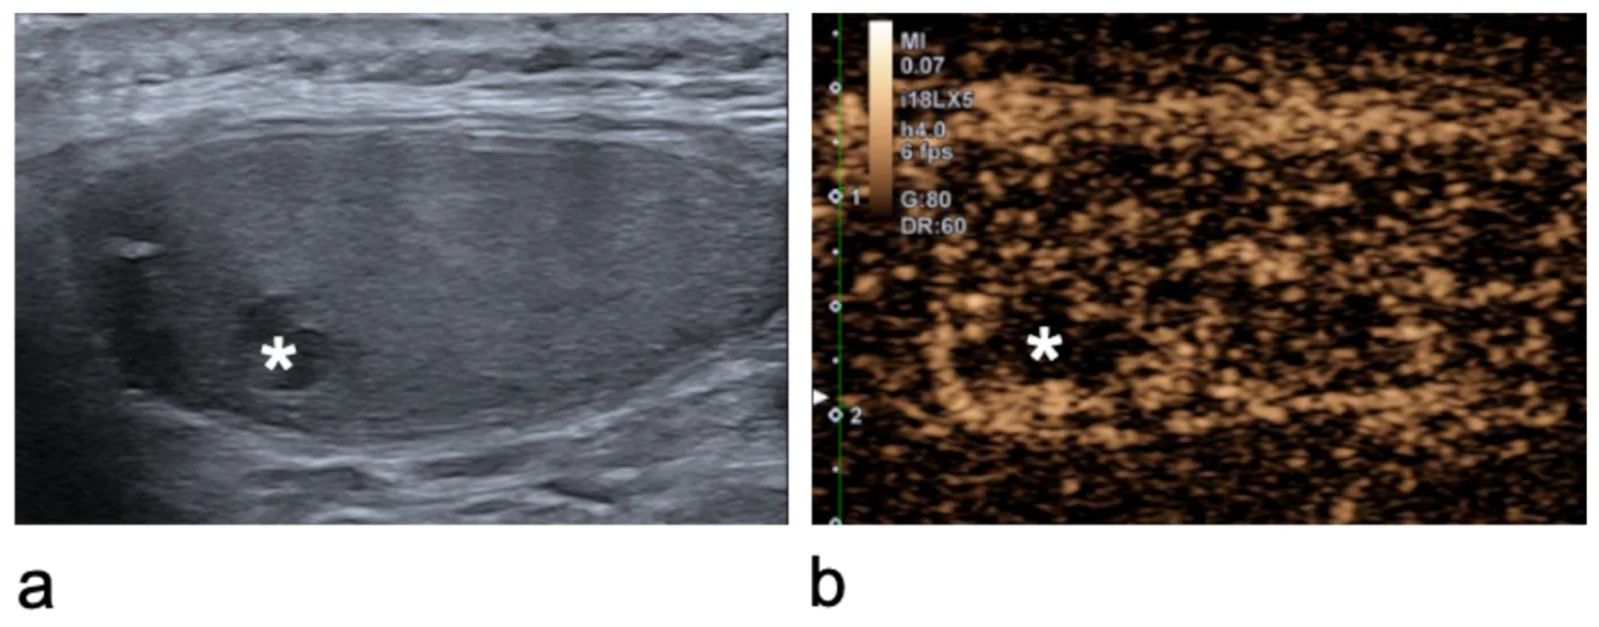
Ultrasound examination performed 40 h after testis-sparing surgery. (a,b) Ultrasound shows a parenchymal haematoma at the site of lesion excision (asterisk). The absence of vascularity of the lesion (asterisk) on CEUS allows differentiation from residual tumour.
